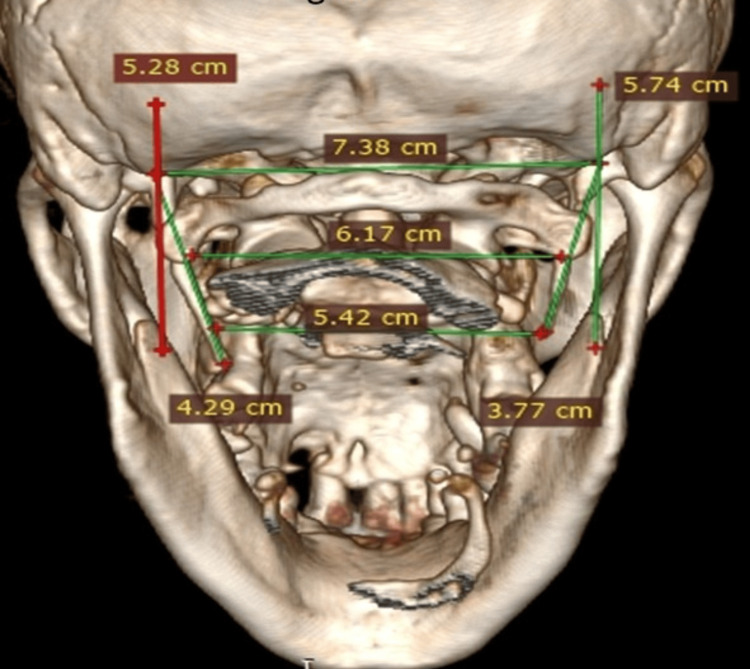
Bilaterally ( >3 cm) elongated styloid process on posterior view of 3D CT. 3D CT, three-dimensional computed tomography.
